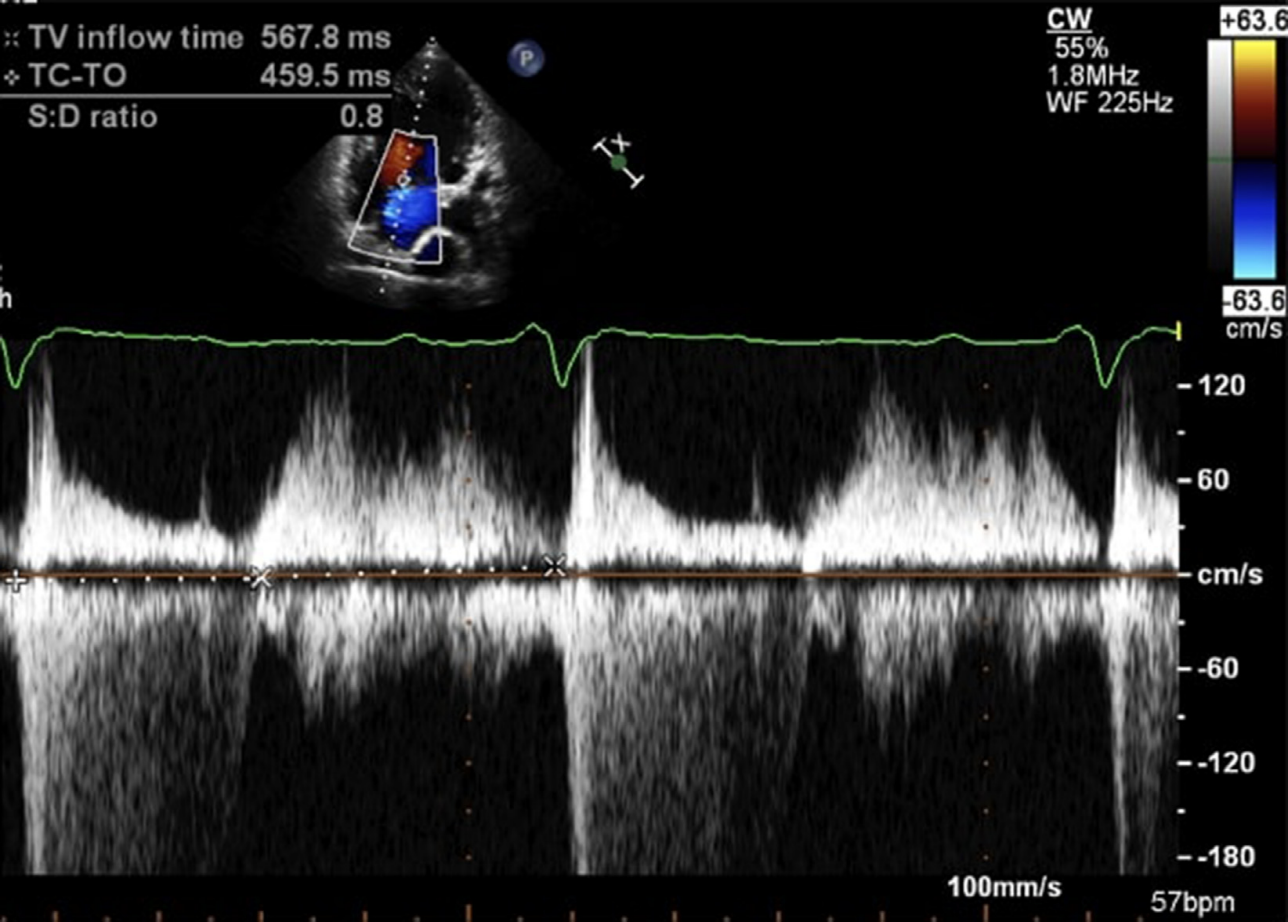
Measurement of the Atrioventricular Systolic to Diastolic Duration Ratio From the Continuous Wave Doppler Signal The atrioventricular systolic to diastolic duration ratio is calculated from the continuous wave Doppler signal of the dominant atrioventricular valve. S:D = systolic to diastolic duration; TC-TO = tricuspid valve closure to tricuspid valve opening; TV = tricuspid valve.
